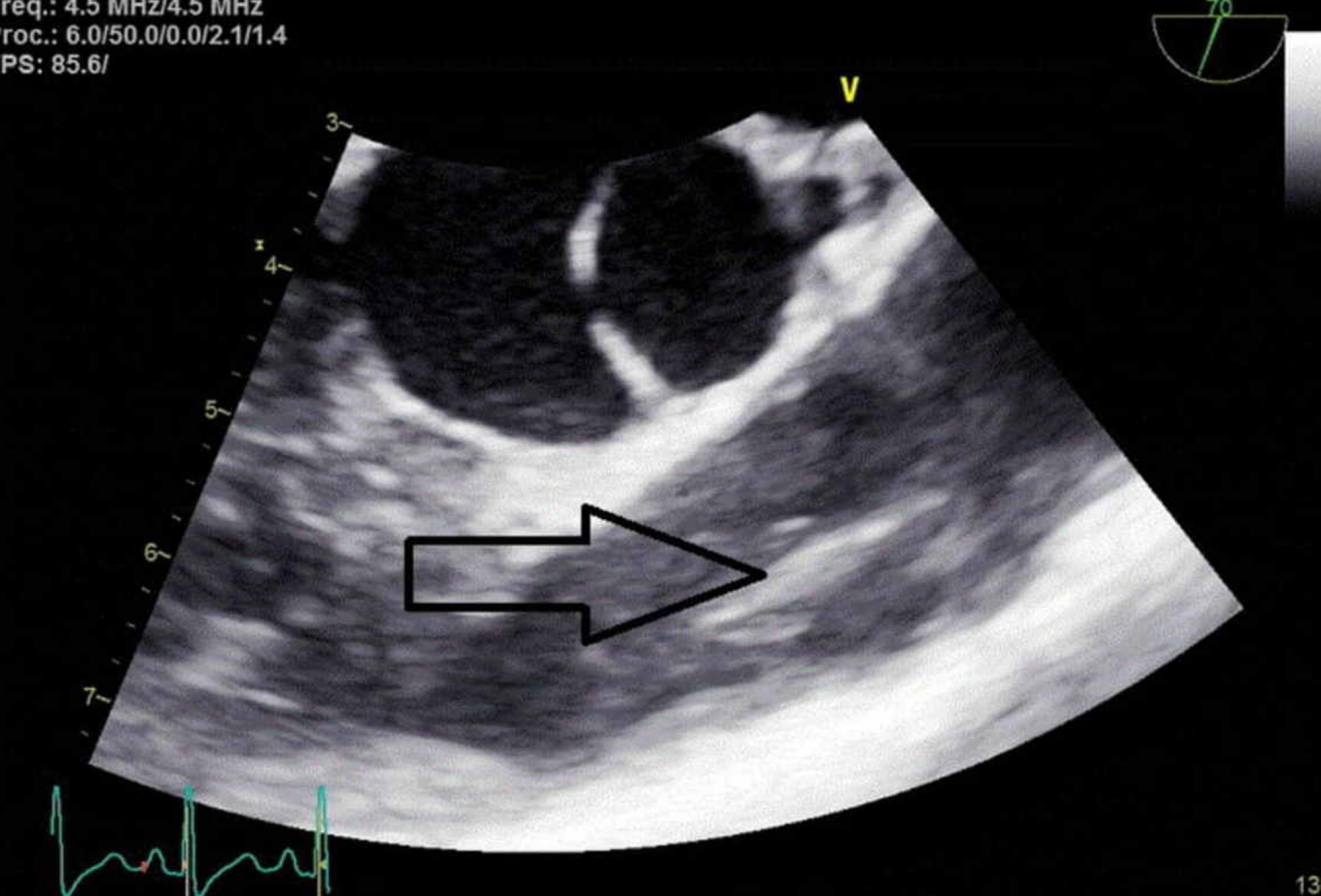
Transesophageal echocardiogram. Pulmonary valve vegetation as shown by the arrowhead.
